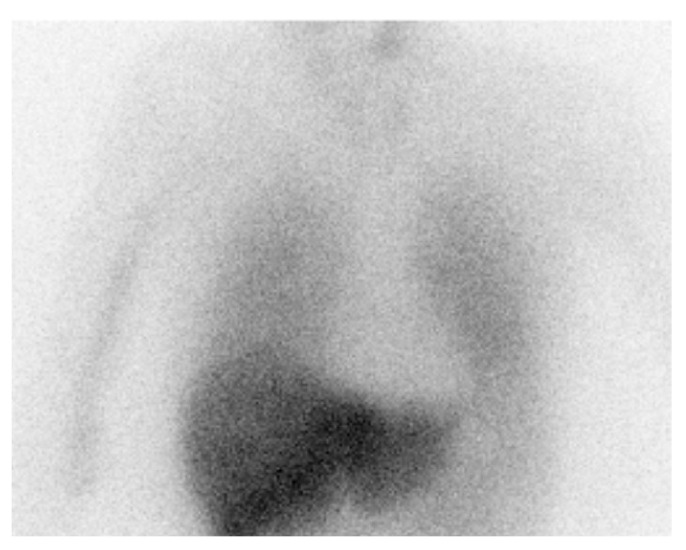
^123^I-MIBG myocardial scintigraphy image taken 3 h after injection. The image shows reduced uptake on ^123^I-MIBG in the heart, indicative of dysfunction in the postganglionic sympathetic cardiac innervation.
